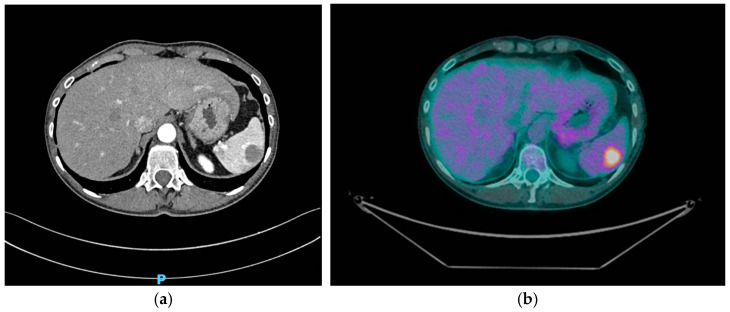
(a) CT scan: round hypodense spleen lesion; (b) PET scan: tracer uptake in the splenic parenchyma.
